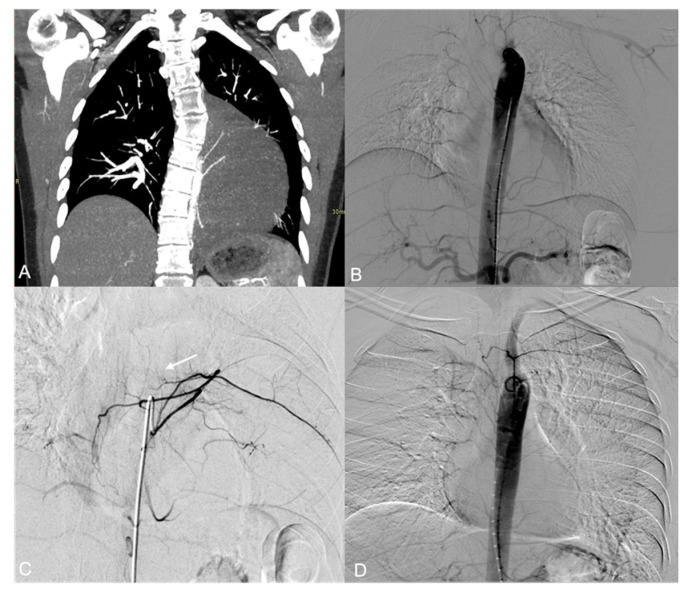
(A) CT Angiography, coronal view: large mass in the posterior left mediastinum dislodging arterial pedicles arising from the thoracic aorta. (B) Preliminary aortography shows the mass has arterial feeders arising mainly from the left intercostal arteries. (C) Selective angiography of the VI intercostal left artery shows the presence of medial radicular branches (arrow). (D) Final aortography after PVA particle embolization.
